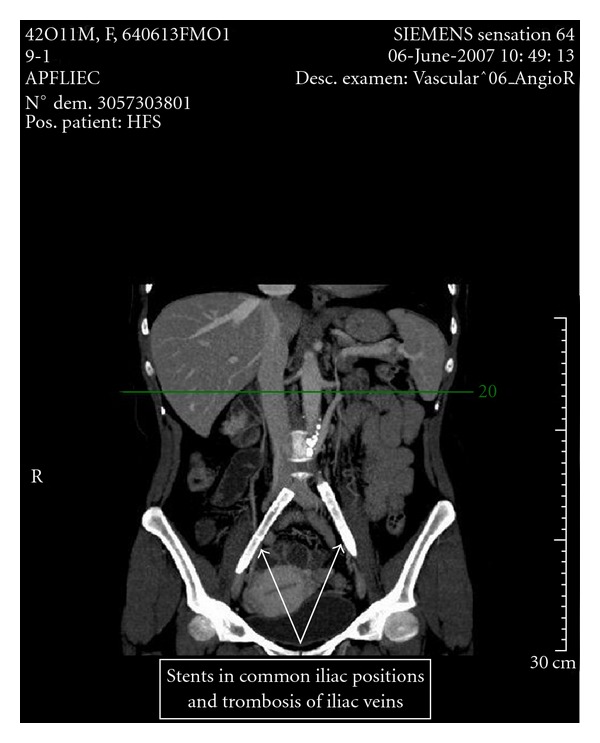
CT angiography image of the receiver pelvic vessels.
